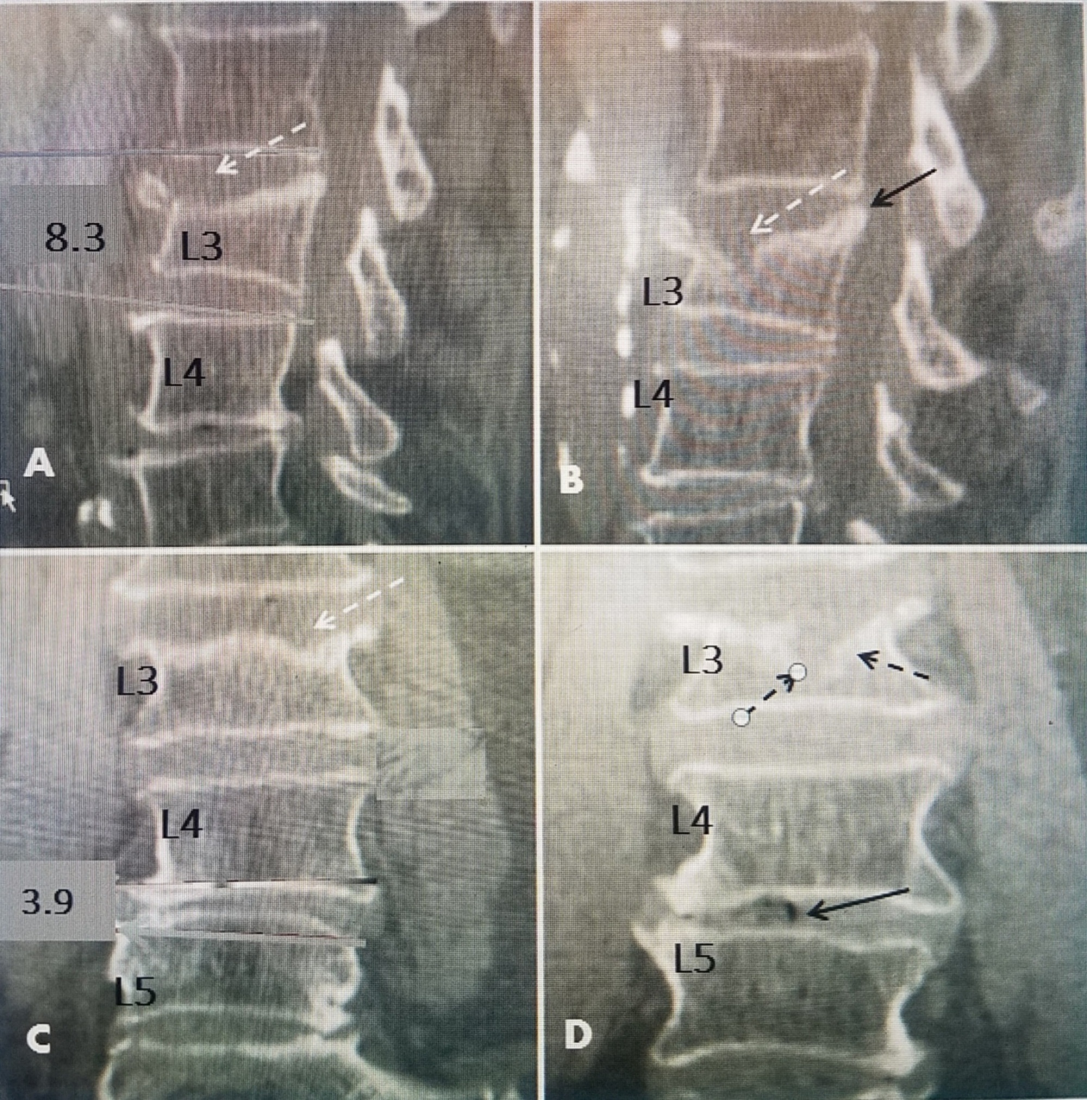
Computerized tomography (CT) scan of a chronic fracture nine months after injury A 76-year-old female with known osteoporosis and bone mineral density (BMD) of -2.3 in the lumbar spine. Initial and follow-up CT scans made nine months after the fall. The patient persisted with chronic mid-lumbar pain despite six months of lumbar bracing. Visual analog score (VAS) of 9. A: Initial CT scan 10 days after fall, showing a 20% anterior superior endplate collapse of L3 (dashed white arrow) with 8.3^0^ of kyphotic angulation and no displacement of the endplate of L3 into the spinal canal. B: Follow-up CT at nine months showing worsening anterior chronic anterior superior endplate compression without the progression of kyphotic angulation at L3. There now is a greater than 50% loss of height of anterior L3 (dashed black arrow). There is a 2 mm posterior displacement of the superior endplate L3 (solid black arrow) and compressed bone surrounding the endplate (dashed black arrow). C: Initial CT scan showing mild-lateral listhesis and 3.9^0 ^of angulation at L4-L5. There are two lateral superior endplate fractures at L3 (dashed white arrow). D: Follow-up CT nine months after onset now showing further collapse but worse laterally toward one side (dashed black arrows). There is the development of vacuum intra-discal changes at L4-L5 (solid black arrow) in the area of lateral listhesis.
